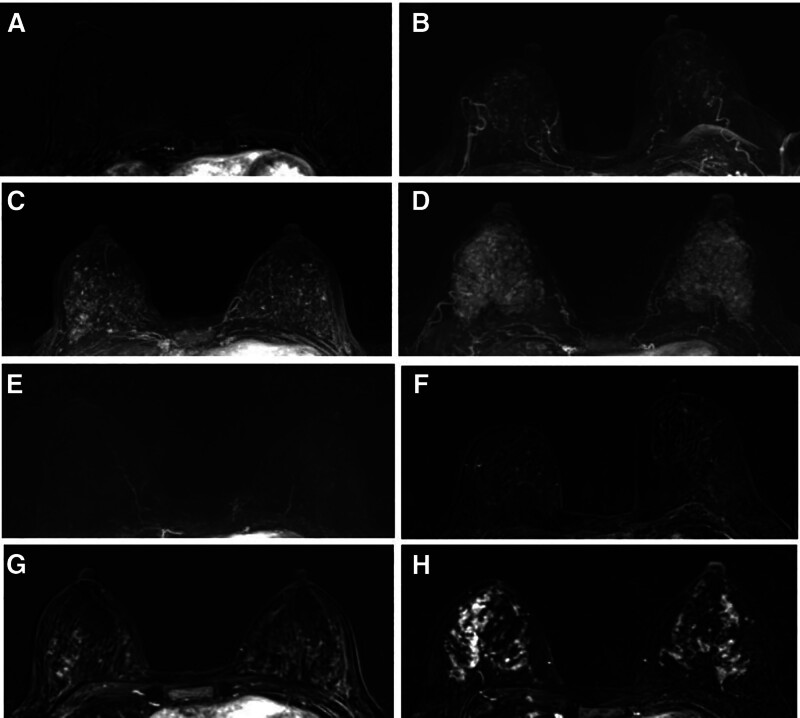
Different categories of BPE images according to MRI scanning. Contrast enhanced MIP subtraction images: (A) minimal; (B) mild; (C) moderate; (D) marked. Subtraction with the mask: (E) minimal; (F) mild; (G) moderate; (H) marked. BPE = background parenchymal enhancement, MIP = maximum intensity projection, MRI = magnetic resonance imaging.
